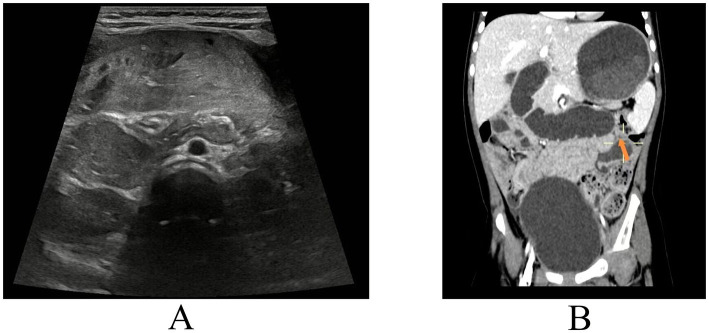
(A) Gastrointestinal ultrasound suggesting duodenal dilatation. (B) Abdominal CT suggesting high-grade incomplete mechanical intestinal obstruction, possibly caused by a foreign body or space-occupying lesion (indicated by the yellow arrow).
